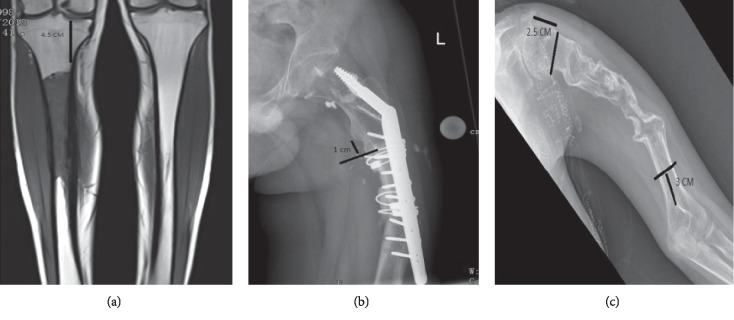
(a) MRI of right tibia of patient with a tibial osteosarcoma, with 4.5 cm remaining length for anchorage of JSE after resection of the tumor with negative margin. (b) Left proximal femur X-ray of a failed bone allograft and DHS cut-off, with 1 cm of cortical bone remaining below the lesser trochanter. A JSE can be used here to revise this failed bone allograft. (c) Left humerus X-ray of postchemotherapy humerus osteosarcoma, showing a 3 cm of cortical bone remaining above the olecranon fossa which makes the sparing of the elbow joint possible. Furthermore, 2.5 cm of bone is remaining proximally making the sparing of the glenohumeral joint possible.
